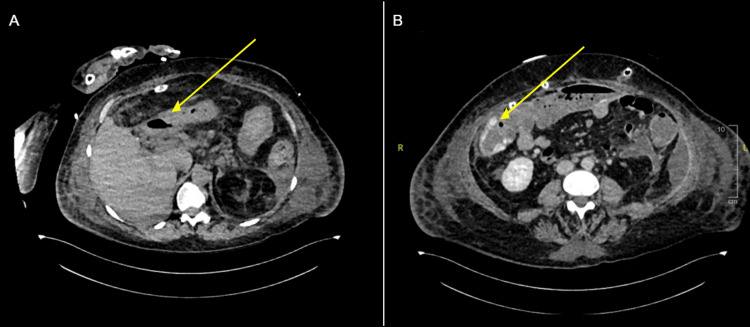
Computed tomography (CT) angiogram abdomen in axial view, demonstrating pneumoperitoneum arising from the caecum (A, yellow arrow), and active brush contract (B, yellow arrow).
